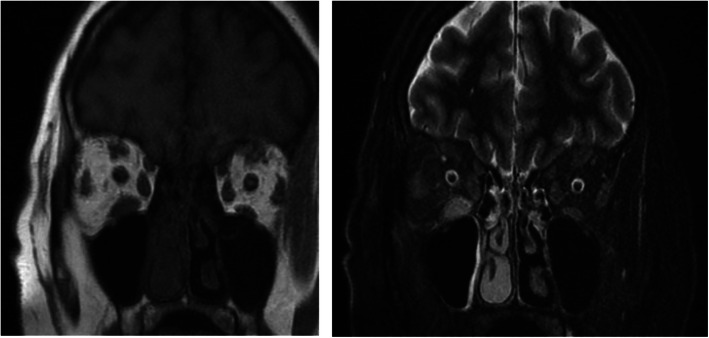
Orbital MRI at presentation showing tumefaction and edema of the extraocular muscles of the orbit (right inferior and right lateral muscle) with inflammation of the periorbital soft tissue
